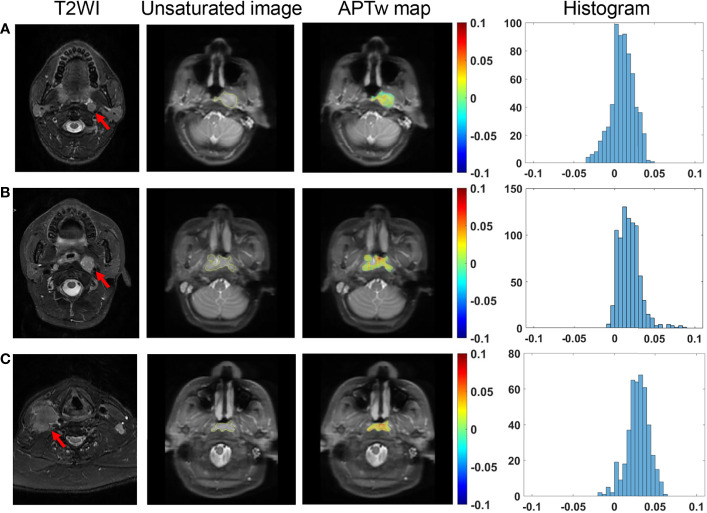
Comparison of T2-weighted images of metastatic lymph nodes (red arrows), and unsaturated S0 images with ROI overlaid, APT_w_ maps and histograms in NPC tissues among different N stages. A 39-year-old male patient with N1 stage NPC (A), a 33-year-old female patient with N2 stage NPC (B), and a 65-year-old male patient with N3 stage NPC (C).
